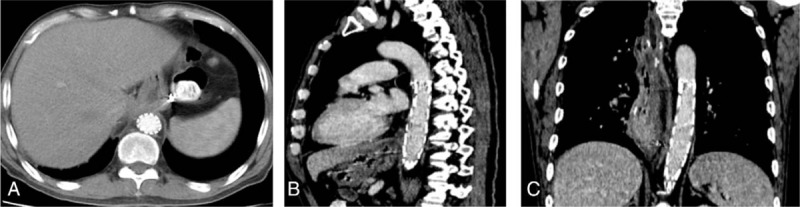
Contrast-enhanced CT showing no evidence of extravasation. CT = computed tomography.
